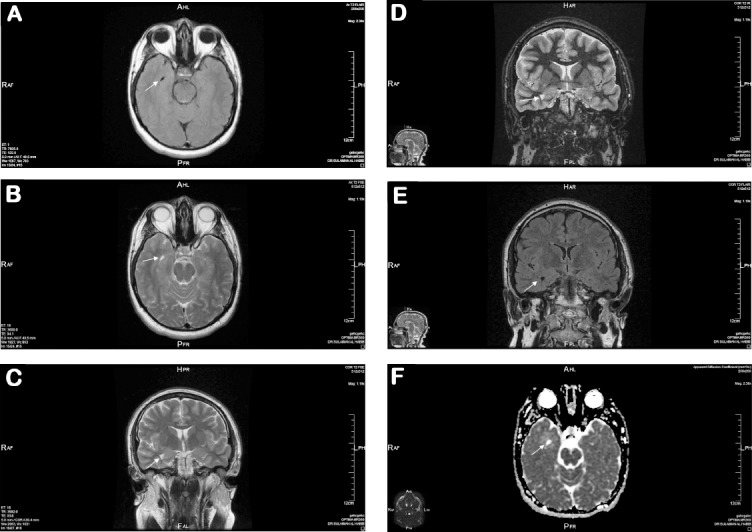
Showing right temporal trophic changes with dilated right temporal horn a) FLAIR axial MRI, b) T2 FSE weighted axial MRI, c) T2 FSE weighted coronal MRI, d) T2 IR weighted coronal MRI, e) FLAIR coronal MRI, f) Diffusion weighted coronal MRI.
